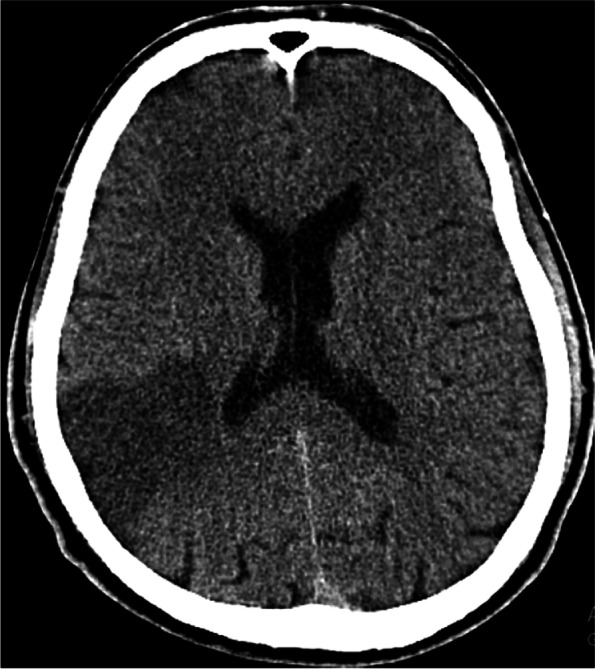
Brain CT scan on admission showed the right parietotemporal lobe infarct
